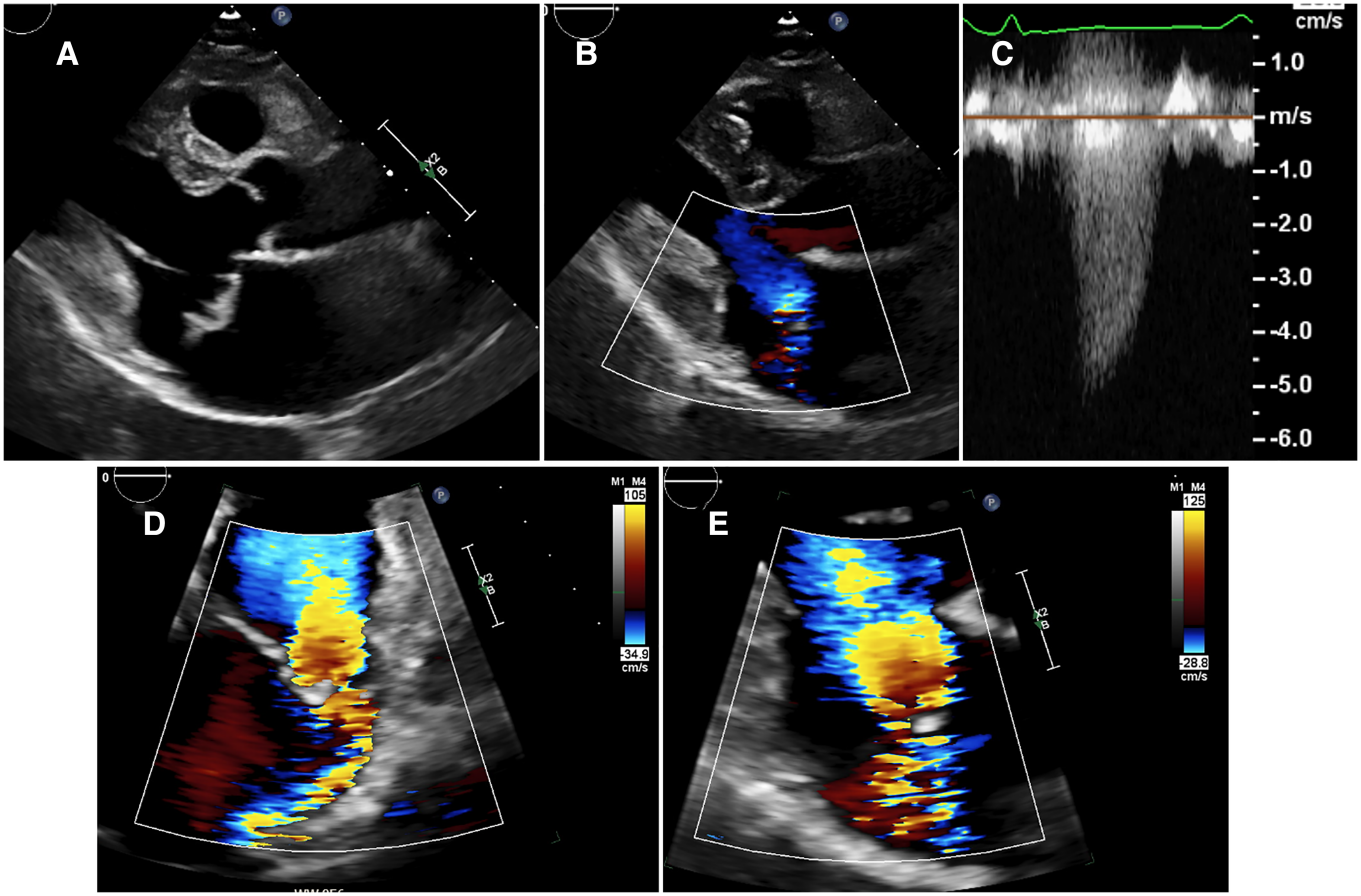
Patient with primary MR with typical features of Barlow's disease. Presence of a MV valve prolapse (A3P3) with mitral annulus dilatation and disjunction (A). The MR jet is very eccentric with small color jet size (B). The best CWD MR envelope is obtained in the long-axis parasternal view, revealing a mid-late systolic MR (C). Using the PISA method, the proximal flow convergence area displays an oblong shape in apical-4-chamber view because being constrained by the LV lateral wall (D). The proximal flow field is better delineated in long-axis parasternal view (E), with an “urchinoid” shape because of loss of Doppler signal on its angles. CWD, Continuous-Wave Doppler; LV, left ventricular; MR, mitral regurgitation; MV, mitral valve; PISA, proximal isovelocity surface area.
